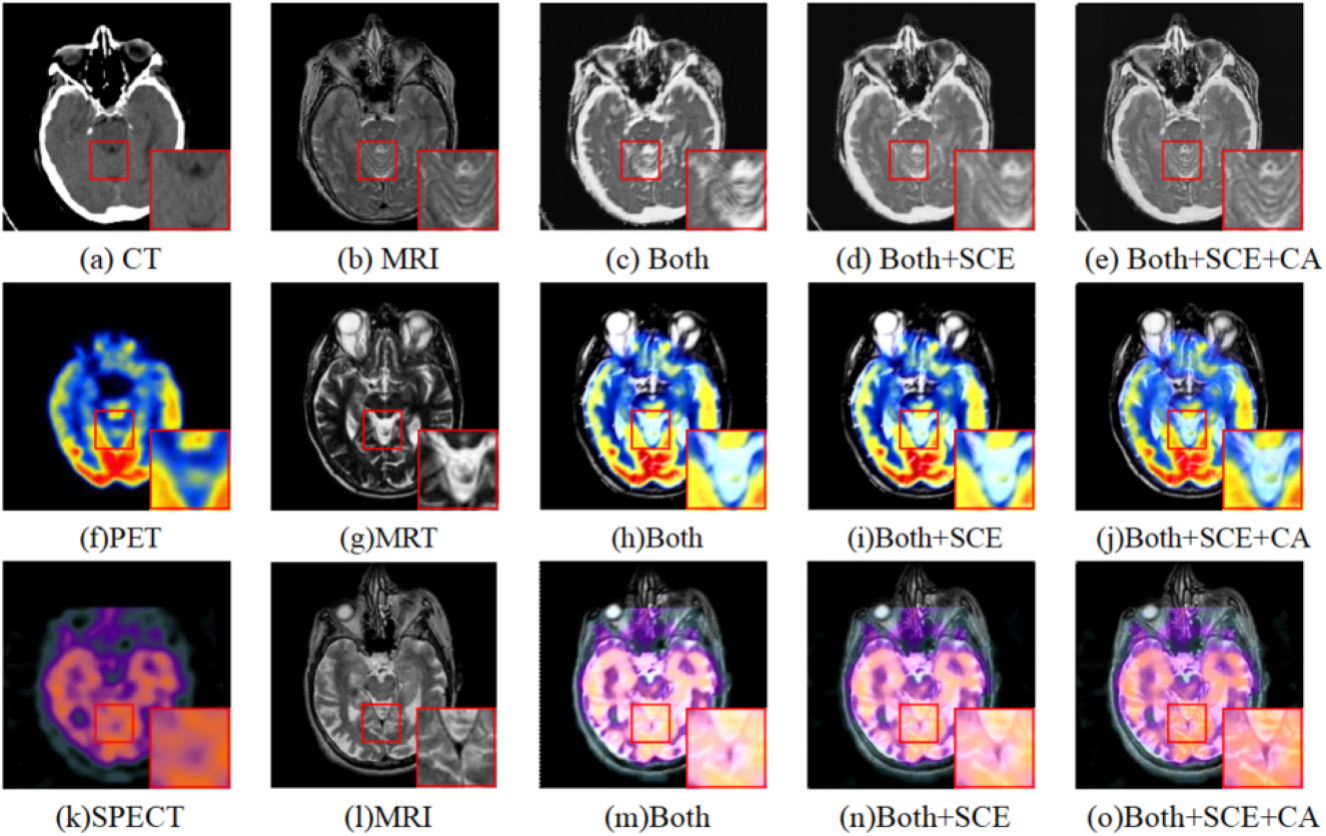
Fused images of computed tomography-magnetic resonance imaging (CT-MRI), positron emission tomography-magnetic resonance imaging (PET-MRI), and single-photon emission computed tomography-magnetic resonance imaging (SPECT-MRI) using different modules.
